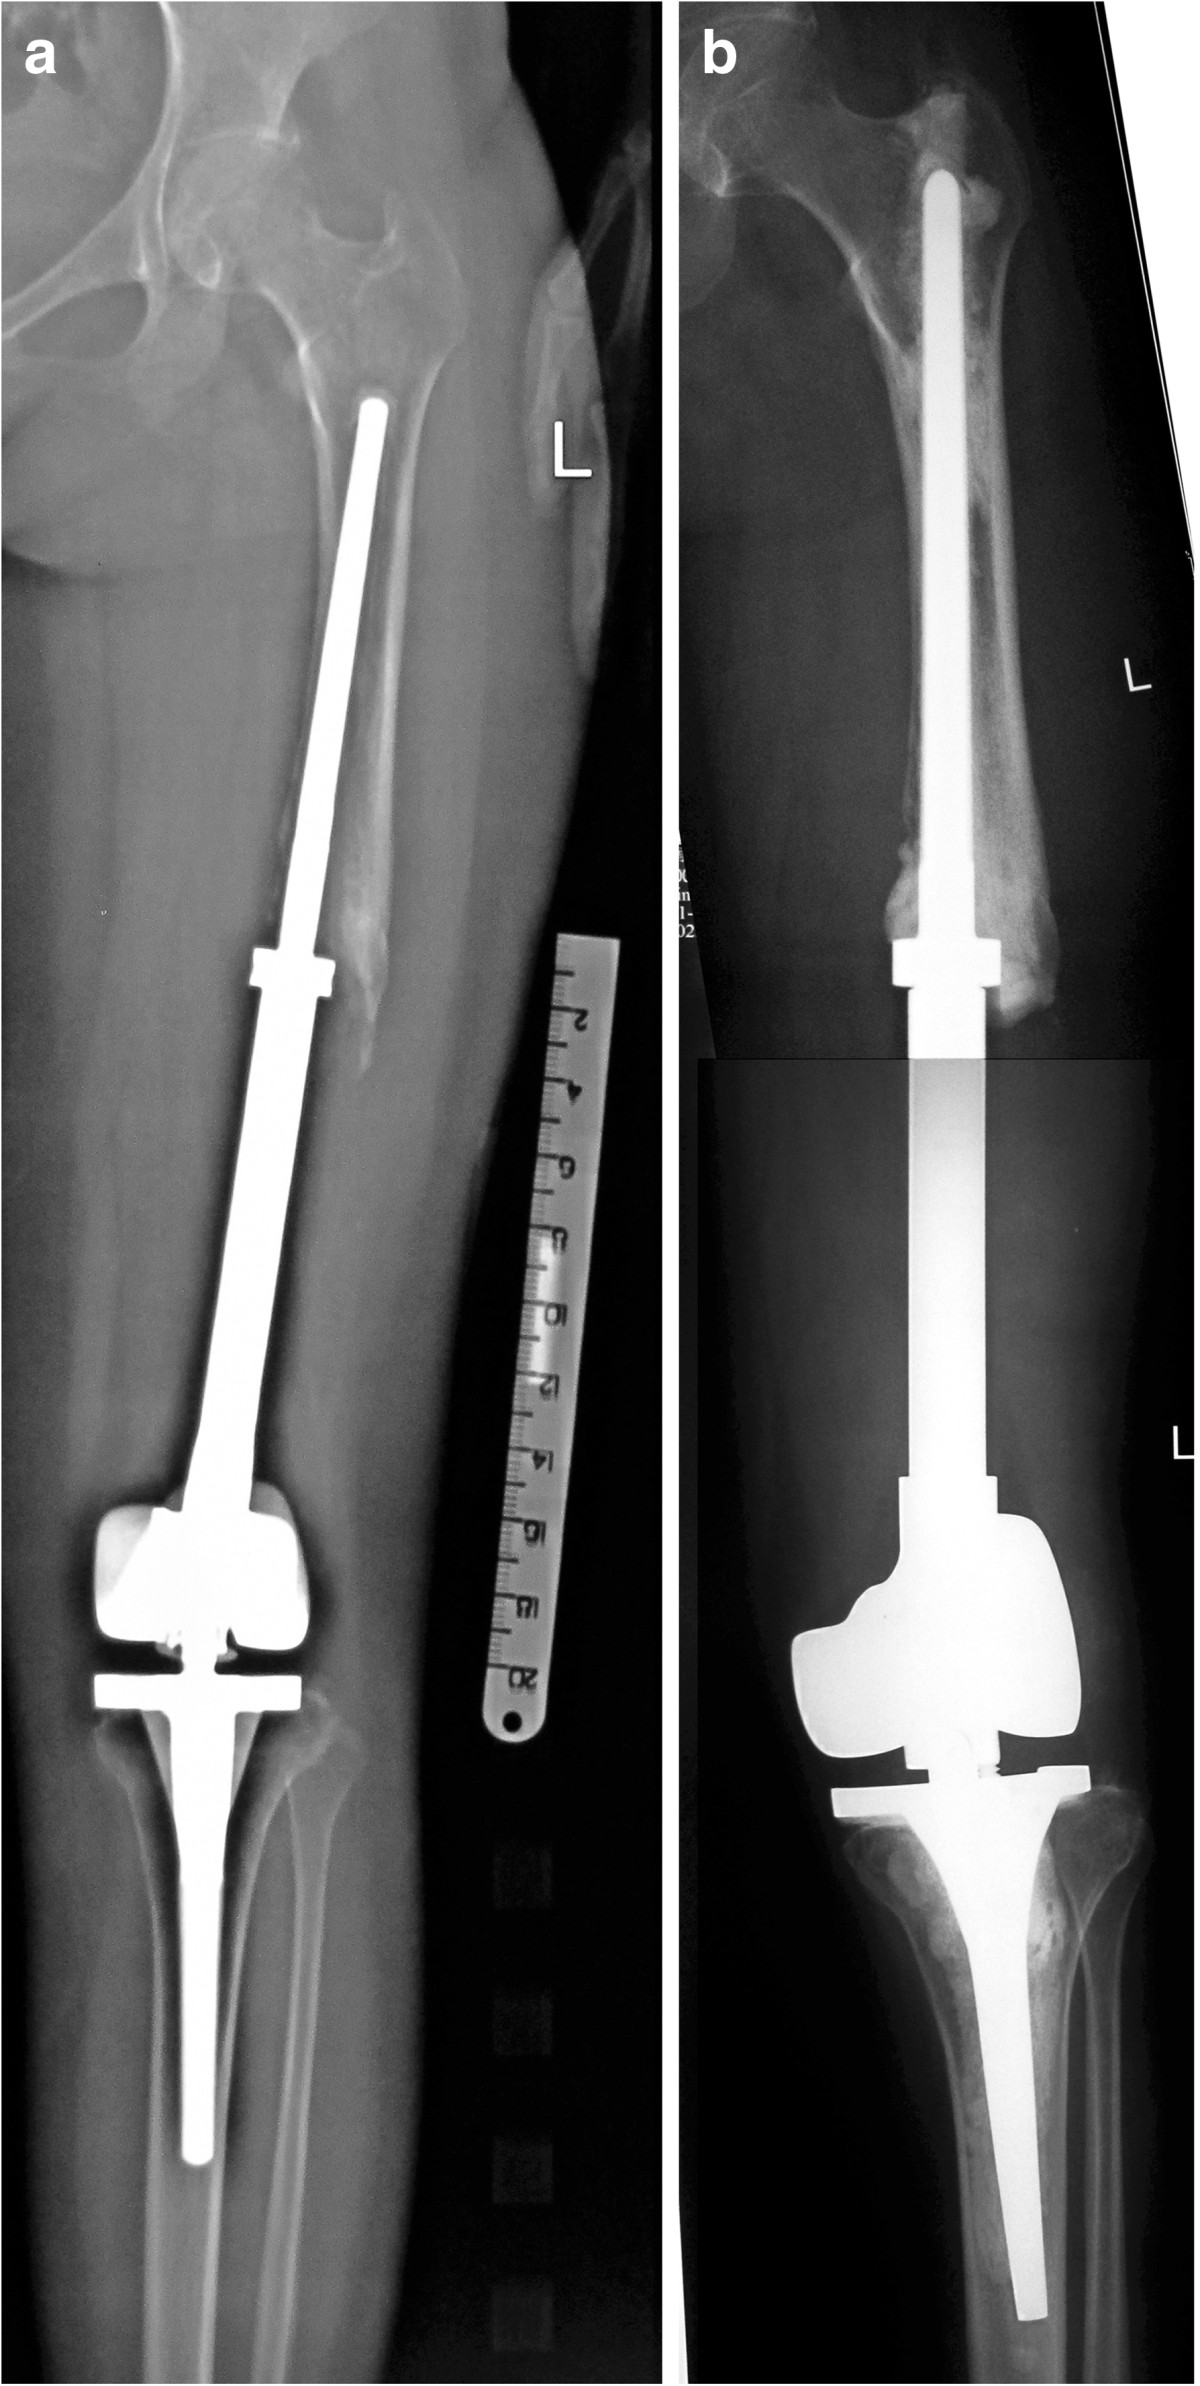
A 17-year-old female patient with osteosarcoma in left distal femur was treated with prosthesis replacement. a 8 years after operation, bone lose and prosthesis loosening could be found on X-ray. Physical examination demonstrated 6 cm limb length discrepancy with 26 in MSTS score and 44 in QOL. b After prosthesis revision, the left lower limb alignment restored and 3 cm limb length discrepancy remained.
